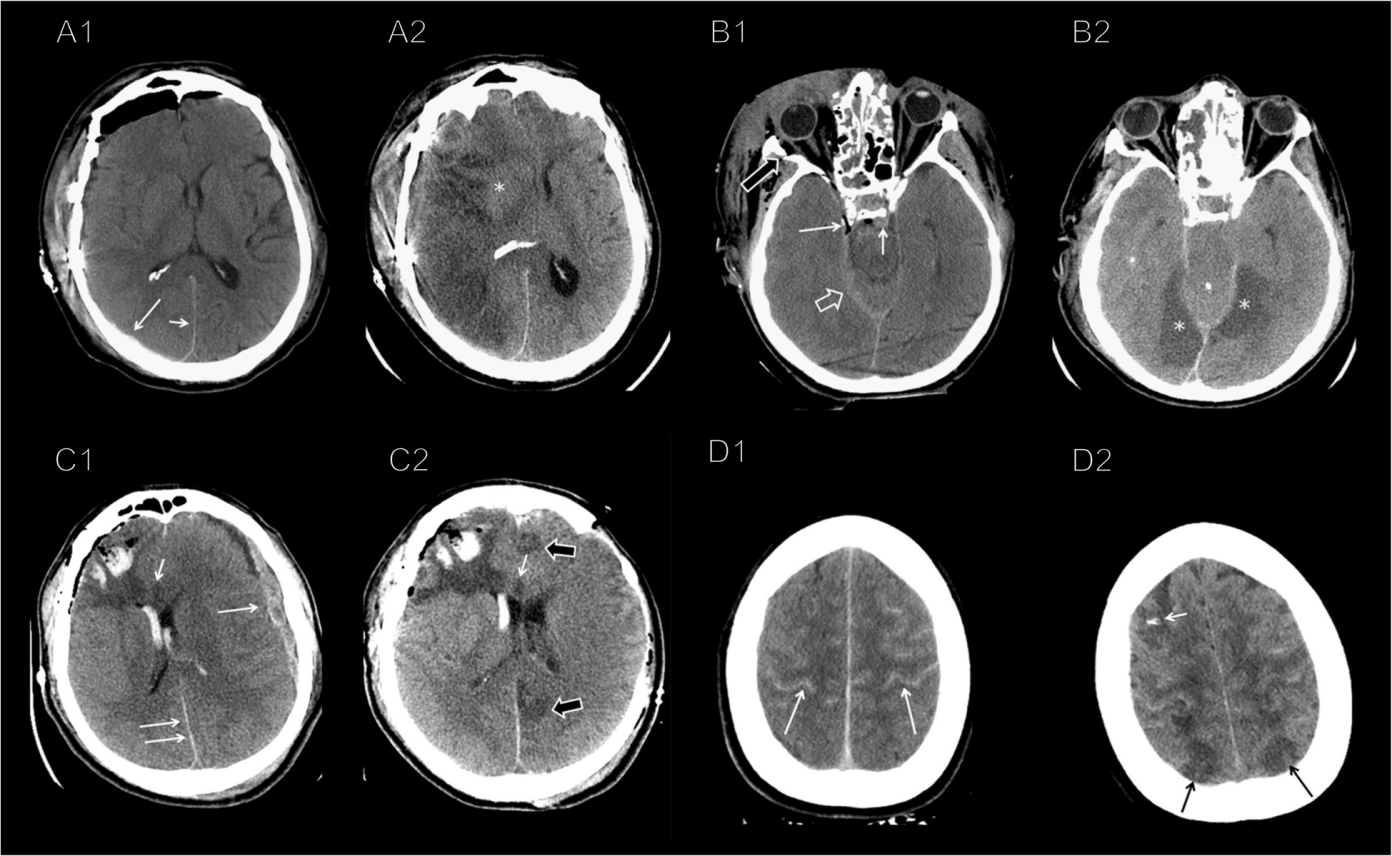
CT scan showing posttraumatic cerebral infarction (PTCI). A1 MCA PTCI: acute parietal subdural hematoma on the right side (long arrow), extending to the falx (short arrow). A2 CT scan 9 days later showed an *acute ischemic lesion in the superficial territory of the right MCA* (preserved right lenticular nucleus, white *). B2 PCA PTCI: acute subdural hematoma along the right side of the tentorium (empty arrow), extra-axial blood in the prepontine cistern (short arrow), and small para sellar bubble air (long arrow) on admission brain CT. B2 Brain CT scan at 15 days showed complete effacement of the basal cisterns and bilateral temporo-occipital hypodensities (*), consistent with *acute ischemic lesions in the territory of both PCA*. C1 ACA PTCI: hemorrhagic contusions of the right frontal lobe mixed with air and perilesional vasogenic edema, intraventricular hemorrhage, a thick left frontoparietal acute subdural hematoma (long arrow) with midline shift to the right, and a thin acute subdural hematoma along the posterior falx (double arrows). C2 Left frontoparietal craniectomy and hematoma evacuation showed multifocal hypodensities in the anterior and posterior portion of the left cingulate gyrus (white outlined arrows), consistent with *acute ischemic lesions in the territory of the left ACA*. The small hypodensity in the genu of the corpus callosum (short arrow), barely visible in the first exam, is consistent with a shear-strain injury. D1 Superficial watershed PTCI: thick acute subdural hematomas along the whole tentorium and the left frontotemporal convexity. Diffuse subarachnoid hemorrhage is also visible at the vertex (long white arrows). D2 Bilateral cortical hypodensities in the posterior parasagittal regions (black arrows), consistent with *acute watershed ischemia at the boundary zone between the MCA and ACA territories*. Note the probe for the intracranial pressure monitoring in the left frontal lobe (short white arrow)
